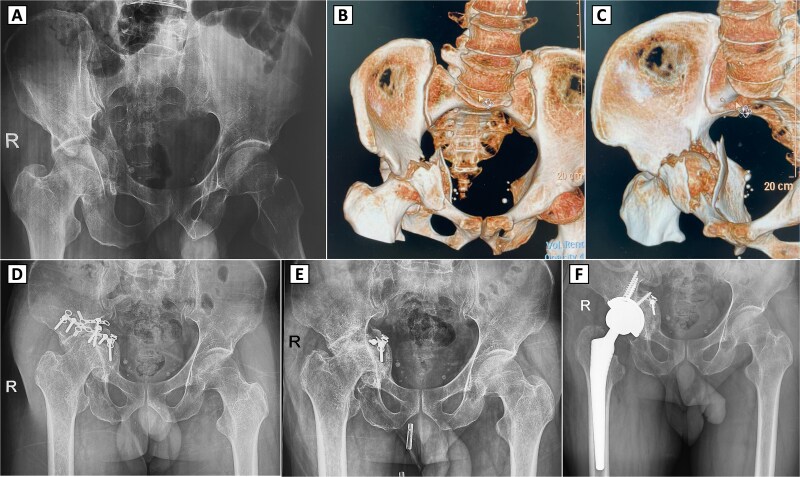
Preoperative imaging, 3D CT reconstruction, and postoperative radiographs with complex right acetabular fracture and subsequent surgical interventions. (A) Preoperative anteroposterior (AP) pelvic X-ray demonstrating a displaced right acetabular fracture with protrusion of the femoral head into the pelvis. (B, C) 3D computed tomography (CT) reconstructions (anterior and superior views, respectively) revealing the comminuted acetabular fracture involving the posterior column and posterior wall. (D) Postoperative AP pelvic radiograph showing internal fixation of the right acetabulum with reconstruction plates and screws. (E) Radiograph after hardware removal, with partial retention of fixation material, demonstrating secondary osteoarthritis of the right hip joint, including joint space narrowing and femoral head deformity. (F) Postoperative X-ray following the conversion total hip arthroplasty.
